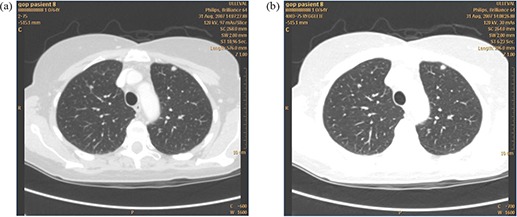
A full‐dose chest CT image (a) in which only standard reconstruction was used; a low dose chest CT image (b)for the same patient in which SharpView postprocessing filter was used.
